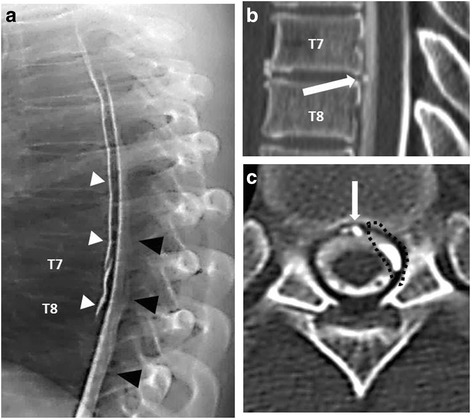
a Lateral dynamic myelographic image demonstrating the site of CSF leakage at T7/T8 (*white arrow*), with contrast medium observed spreading within epidural space (*white arrow heads*) from subarachnoid space (*black arrowheads*). b. Lateral CT myelographic image at T7/T8 demonstrating a partially calcified disc (*white arrow*). c. Axial CT myelographic image at T7/8 level demonstrating a collection of ventral epidural fluid (*black dotted circle*) and a partially calcified disc (*white arrow*)
